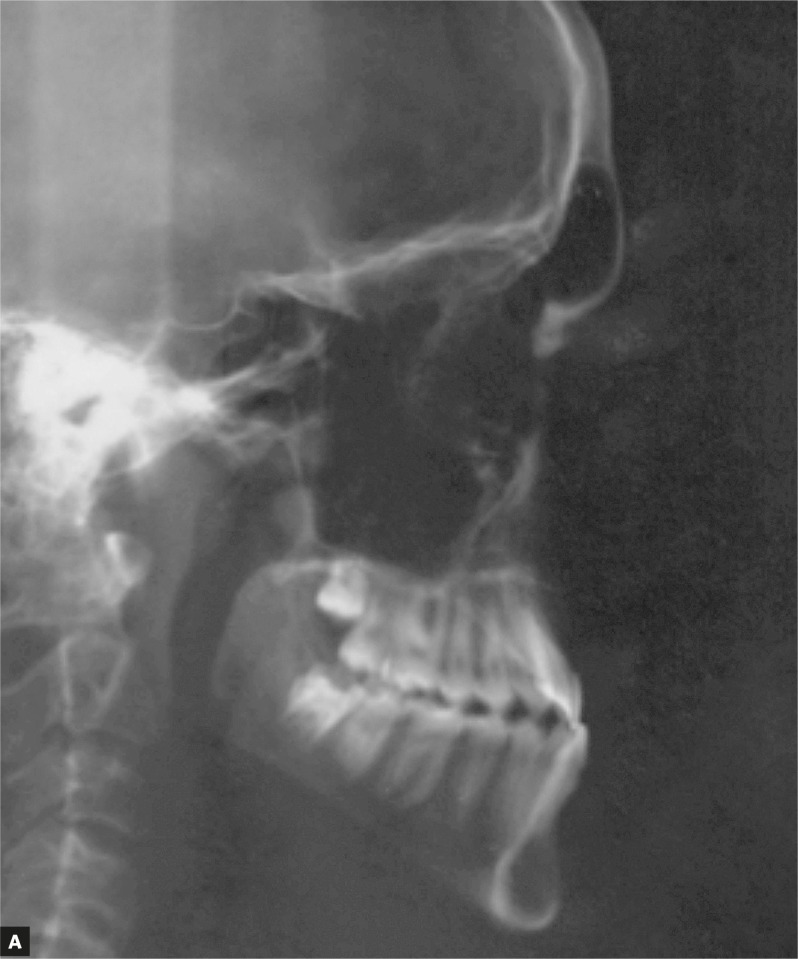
Initial cephalometric profile radiograph (A) and cephalometric tracing (B).
